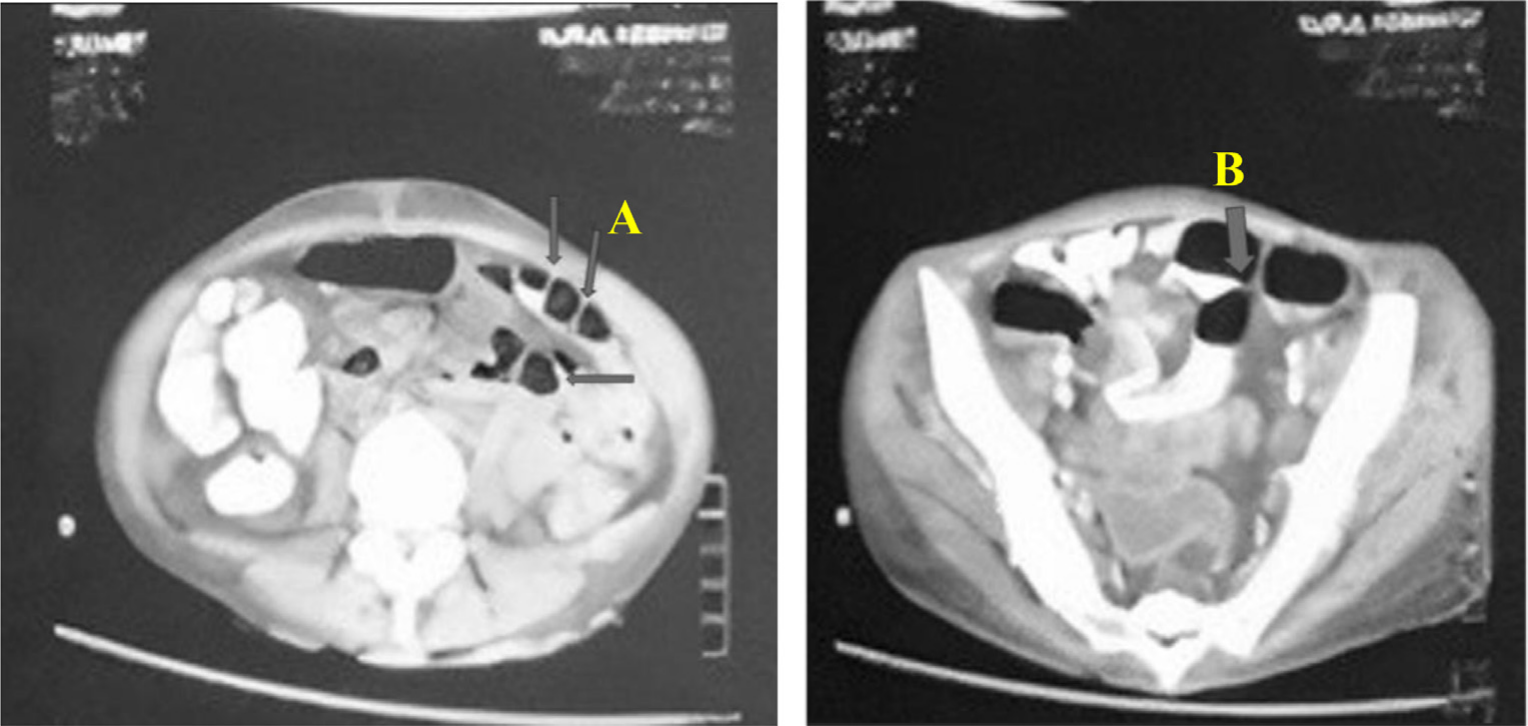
The result of abdominal CT scan on seventh day of hospitalization. (A) diffuse wall thickening and (B) fistula.
